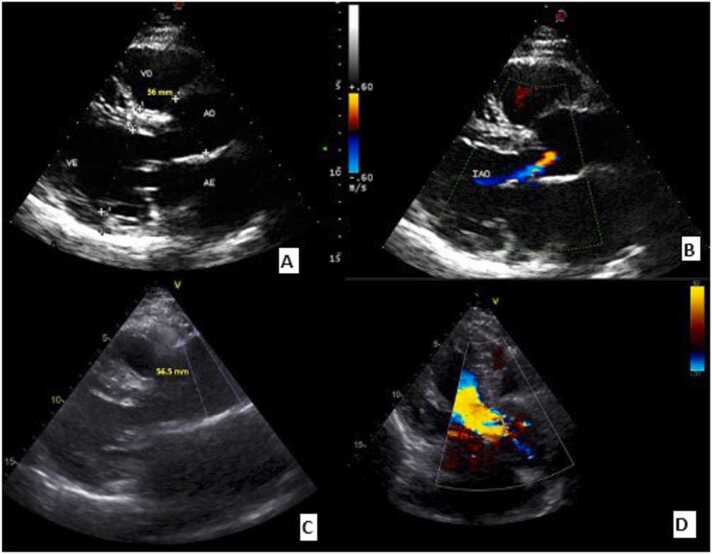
Transthoracic echocardiogram at 2013 (panel A and B) and at 2023 (panel C and D). Panel A depicts the aortic size immediately after heart transplantation (36 mm) and panel B a discrete aortic insufficiency. Panel C shows aortic diameter (56.5 mm) and panel D severe aortic insufficiency at the moment of aortic repair.
